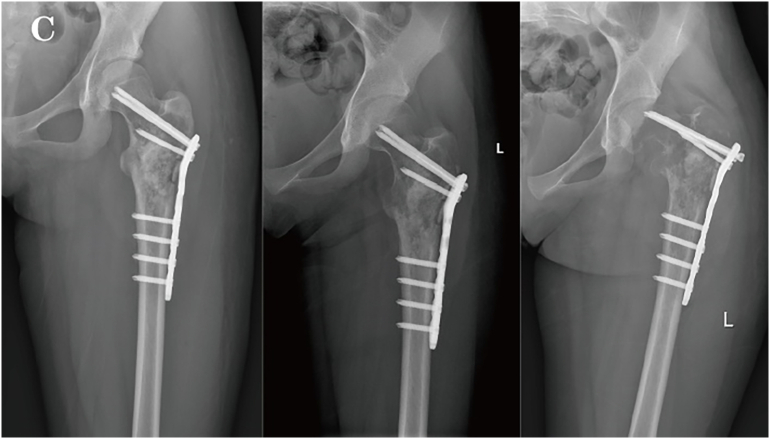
X-ray at two months postoperatively showed hardware displacement and aggressive bone changes.
